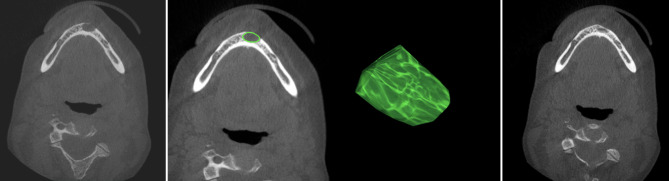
CBCT scans and 3D model of a cyst. CBCT scans: preoperative CBCT of a patient with a suspected radicular cyst (image on the left), one CBCT layer with marked ROI (*in green, left image in the middle*), and a three-dimensional model of the cyst (calculated with OsiriX, right image in the middle). The same patient 6 months postoperatively: the defect has healed almost completely (image on the right).
